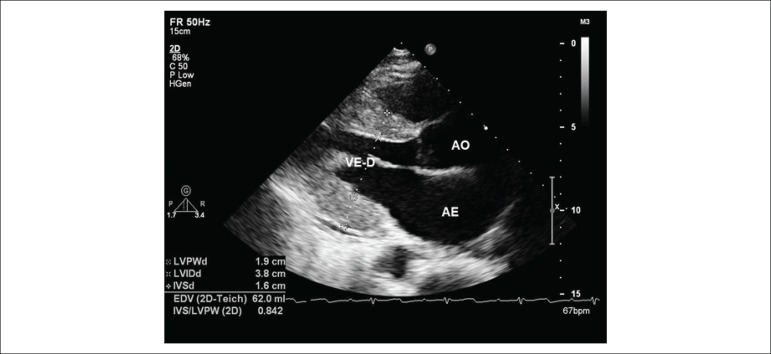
Image obtained from transthoracic echocardiography. Longitudinal parasternal section showing the increased myocardial thickness of the anterior and inferior lateral septal walls of a patient with AL form amyloidosis. Bright aspect of the myocardial walls suggestive of infiltrative disease is observed.
